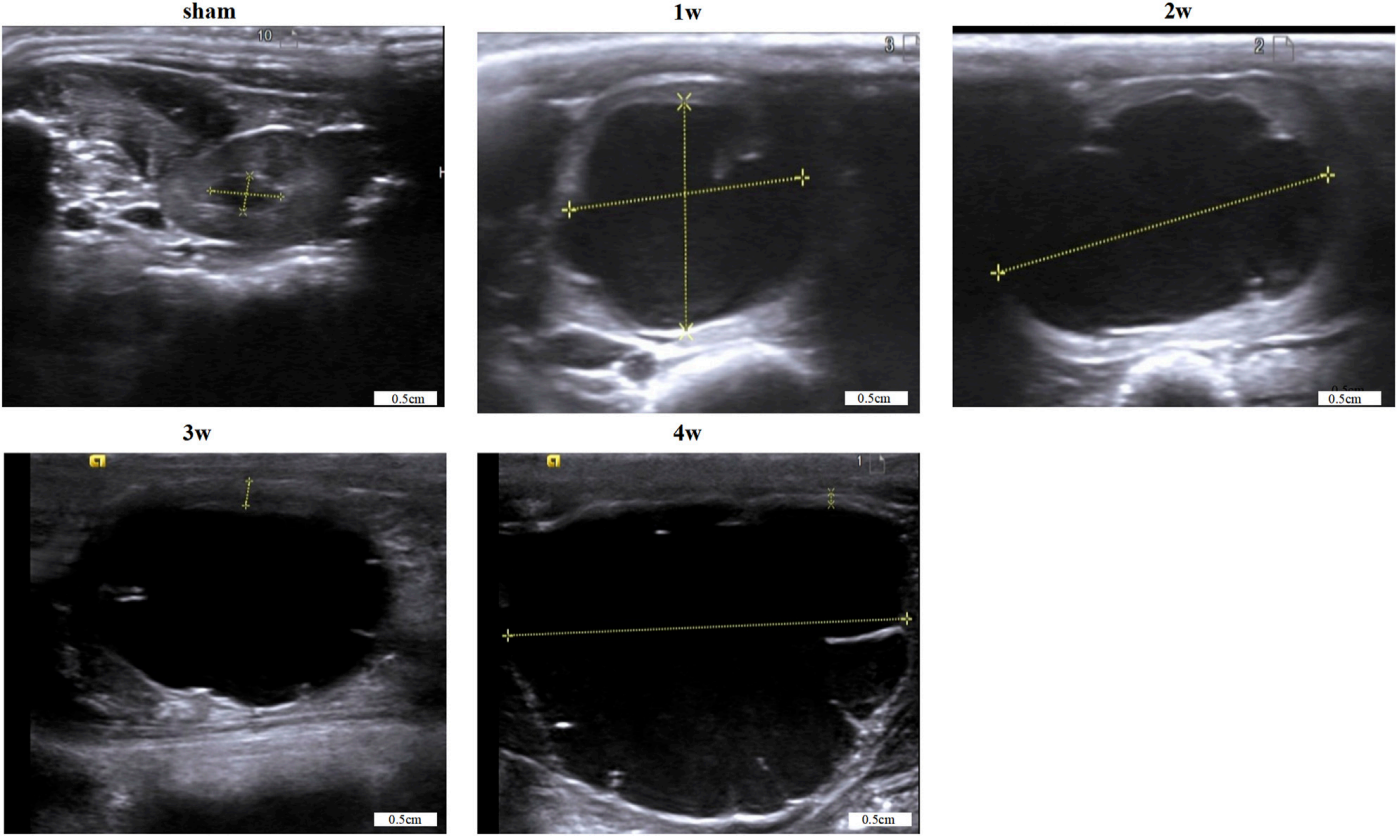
Images of renal pelvis in each group were obtained by B-ultrasound. Scale bars, 0.5 cm.
